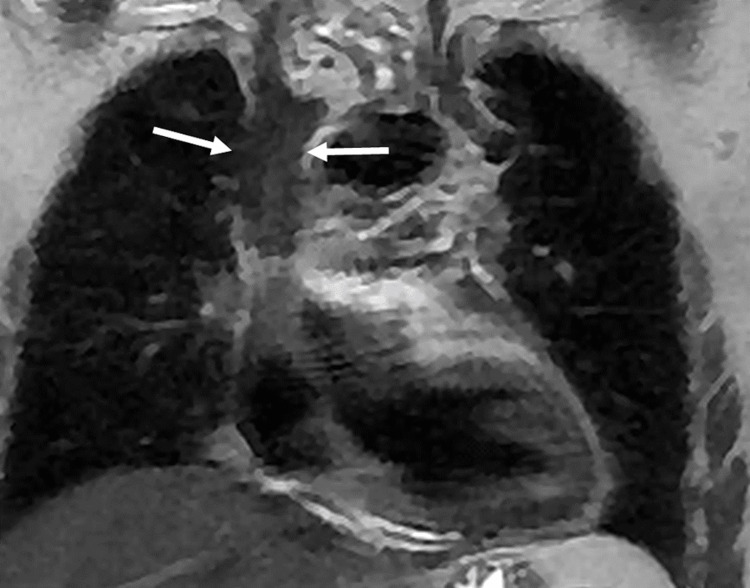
Thoracic MRI in coronal T2 sequence without fat saturation: Extensive parietal thickening of the SVC SVC: superior vena cava
